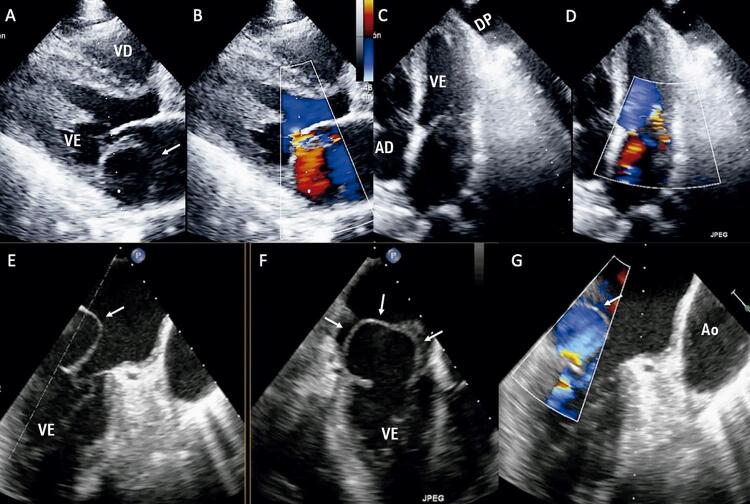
Ecocardiograma com Doppler 2D transtorácico no corte paraesternal eixo longo mostrando átrio de paredes finas tipo cisto (A) e regurgitação mitral moderada (B). Na 4 câmara apical foi visualizado derrame pleural esquerdo (C) e também regurgitação mitral moderada (D). Imagens transesofágicas 2D a 60º e 90º (E, F) confirmaram a presença de uma massa heterogênea tipo cisto (setas brancas) no átrio esquerdo envolvendo o anel mitral posterior. O Doppler colorido revelou fluxo sanguíneo para esta pseudocavidade (G). VE: ventrículo esquerdo; AE: átrio esquerdo; AD: átrio direito; VD: ventrículo direito; Ao: aorta; DP: derrame pleural.
